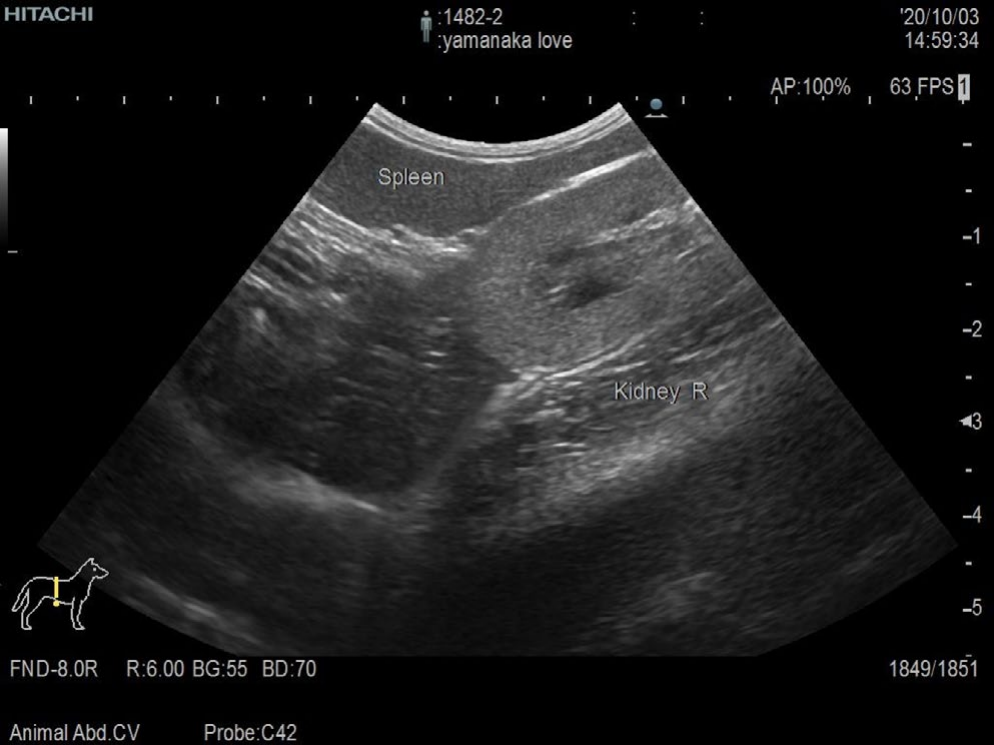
Abdominal ultrasonography findings on the day of surgery. The spleen is in contact with the right kidney and completely displaced to the right side of the abdominal cavity
